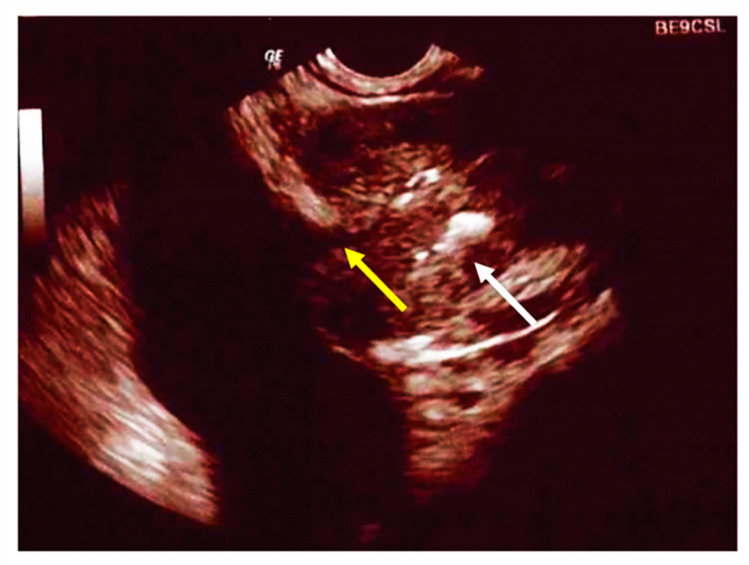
A transrectal ultrasound examination demonstrating calcification within the ejaculatory duct (white arrow) with dilatation of the vas deferens proximally (yellow arrow).
